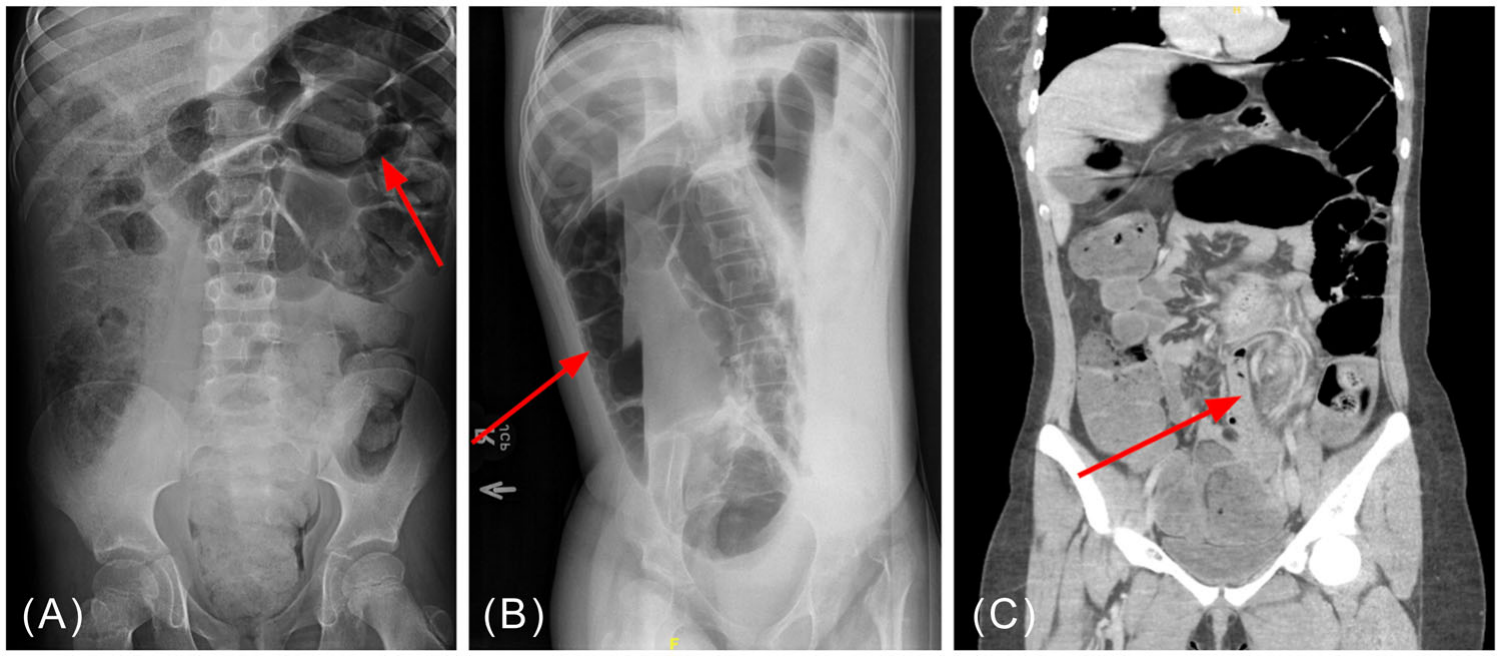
Radiographic images demonstrating classic signs seen in sigmoid volvulus. (A) Patient A's abdominal x‐ray demonstrating dilated colon. (B) Patient B's left lateral decubitus x‐ray demonstrating “coffee bean sign.” (C) Patient C's abdominal computed tomography demonstrating “whirl sign.”
